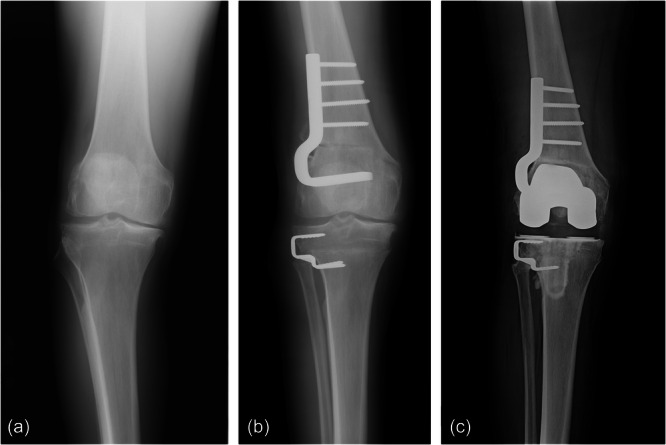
(a and b) Anteroposterior radiographs demonstrating varus knee deformity of the right knee treated with a double‐level osteotomy using a lateral closing‐wedge osteotomy with plate and screws for femoral fixation and a lateral closing‐wedge osteotomy with staples for tibial fixation. (c) Subsequently, after 19.2 years, the patient underwent total knee arthroplasty conversion with a posterior stabilized implant system.
